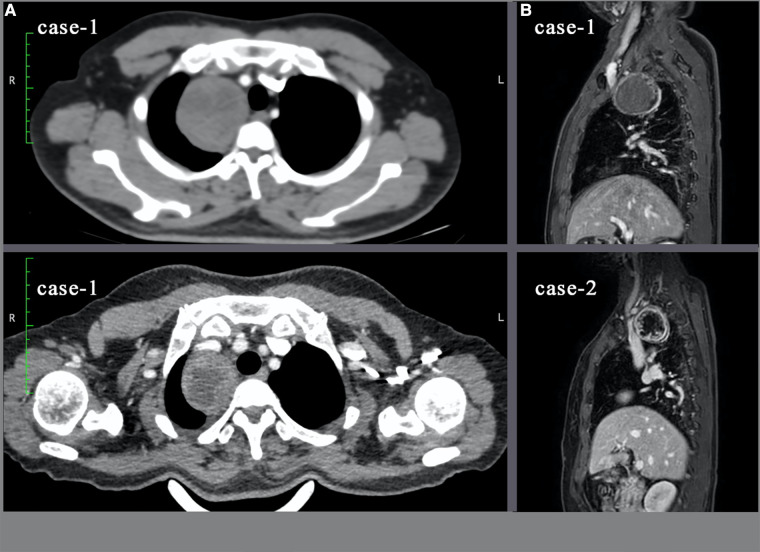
Ct and MRI images of both tumors. (A): Cross-sectional view of the tumor on CT examination; (B): Sagittal view of MRI. In case-2, the tumor has cystic component.
